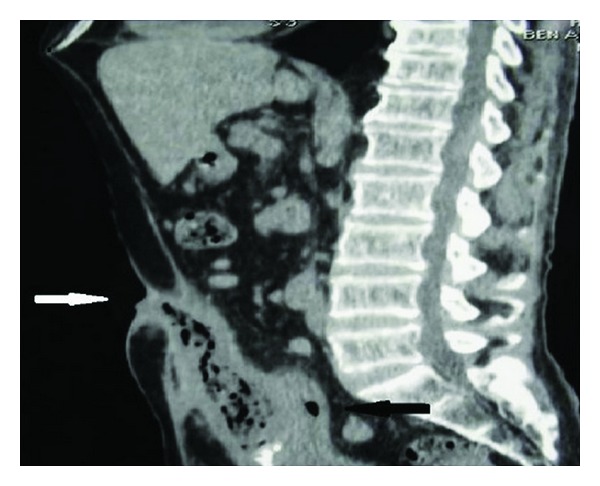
CT reconstruction image revealed a colo-urachal-cutaneous fistula (urachal-cutaneous fistula, white arrow), (colo-urachal communication, black arrow).
